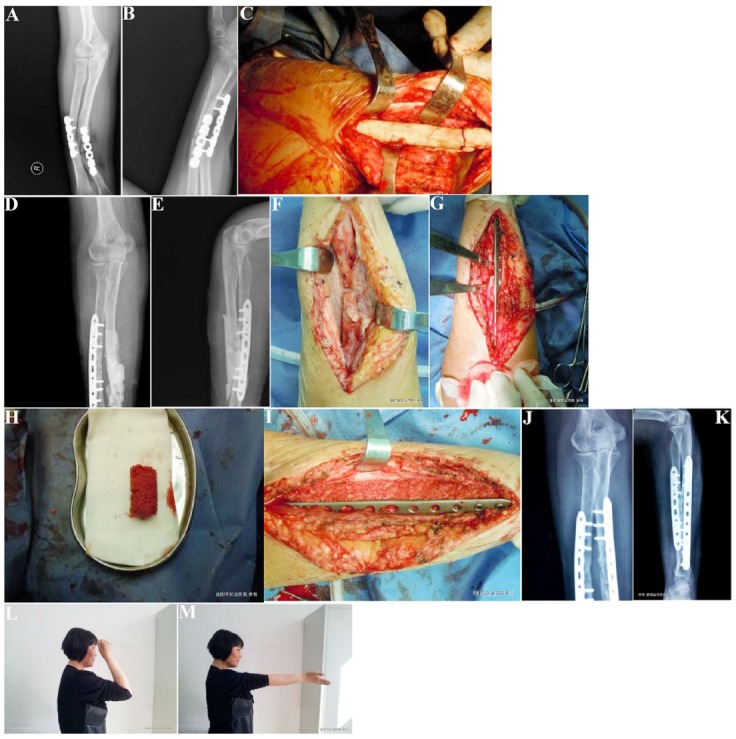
Treatment of a typical case. A female aged 43 years old suffered from open fracture in the right radius and ulna. The patient had repeated redness, swelling and suppuration at the ulnar incision after operation, pain in forearm even after 4 months of dressing changes, deformity and limited function, and the X-rays (A, B) were performed. At stage 1, Debridement was performed at the ulnar fracture end, the antibiotic-loaded bone cement spacer (C) was planted, and the postoperative X-rays (D, E) were conducted. At stage 2, membrane-induced repair of bone defects was performed; the fracture was fixed with steel (F, G); the internal fixation (H, I) of titanium plate was locked; 8 months after operation, the X-ray showed that the infected bone defect was cured (J, K) and the affected limb was well (L, M).
